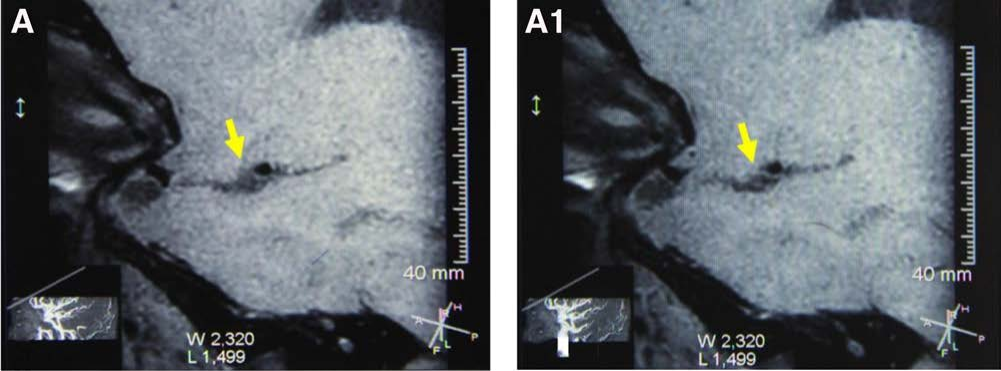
(A and B) HR-MRI showed a circumferential thickening of the wall of the left MCA-M1 segment, and a hypoechoic plaque was visible, accompanied by mild luminal narrowing.
